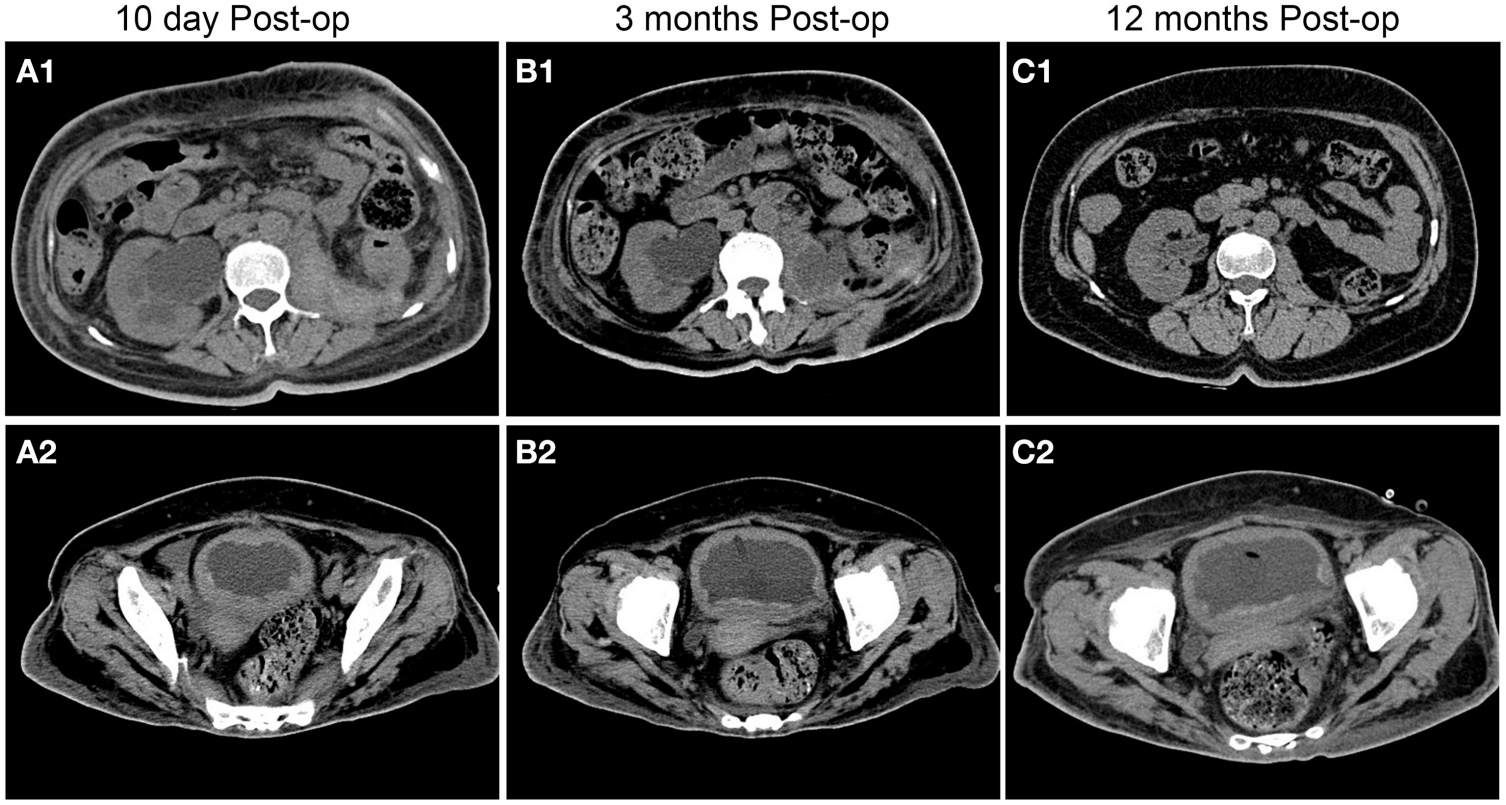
Postoperative follow-up of patients with urinary CT findings. With the extension of time, the right hydronephrosis was gradually relieved, the renal function was gradually improved, and the bladder wall thickening was also significantly improved compared with the preoperative condition.
